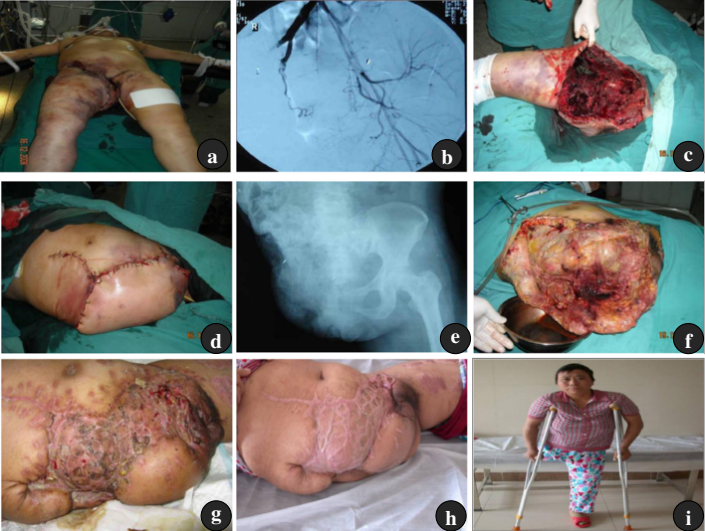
Female, 40 years old, admitted to our hospital 12 h after the right lower extremity was injured by a truck. (a) Resuscitation and the ligation of the external iliac artery were performed in the local hospital. (b) The preoperative angiography showed the external and partial internal iliac artery in the right were occluded, and no bleeding of the artery was observed. (c) The hemipelvectomy was performed 14 h after admission. (d) The wound was closed primarily. (e) Postoperative X-ray imaging. (f) On the third postoperative day, partial necrosis of the skin and severe wound infection was observed during the first debridement. (g) By the second postoperative month, the majority of wound had healed. (h) &(i) By the 12th postoperative month, the wound had healed, and the patient could ambulate with crutches.
